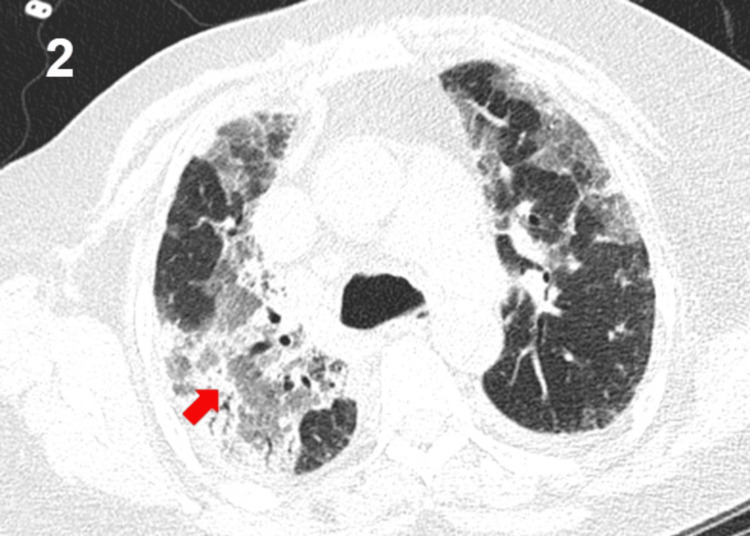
Computed tomography (CT) scan of the chest showing diffuse bilateral involvement with extensive ground-glass opacities and septal thickening, reflecting a generalized interstitial-alveolar pattern (red arrow). The extent and distribution of these findings correlate with severe hypoxemic respiratory failure and are characteristic of drug-induced pneumonitis in the appropriate clinical context.
